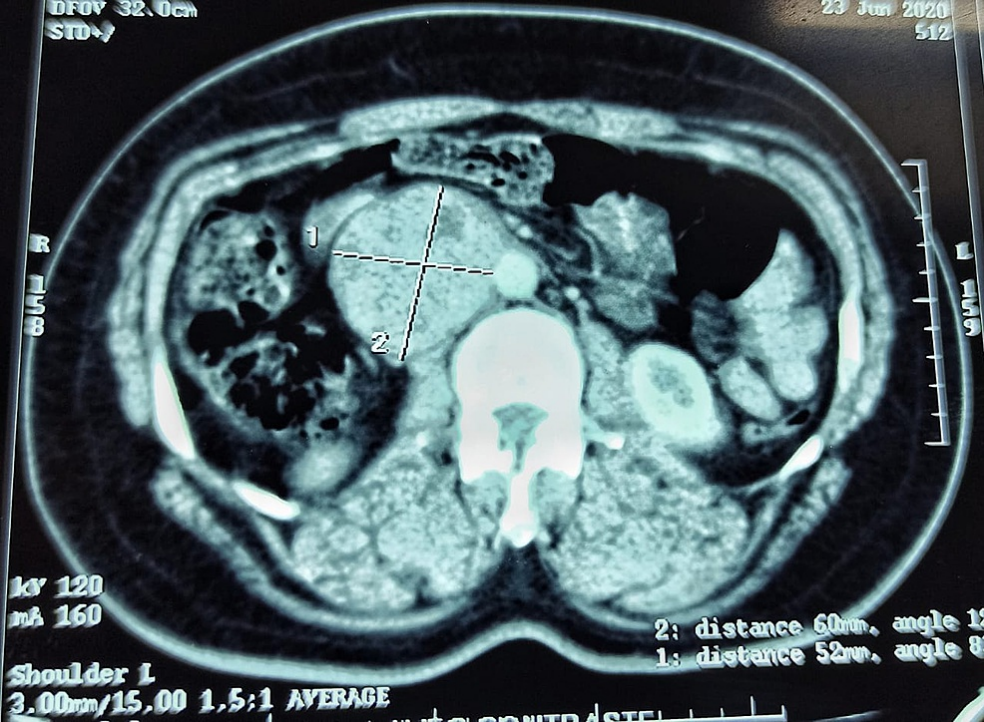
Axial CT of the abdomen reveals a retroperitoneal mass enclosing the inferior vena cava.
